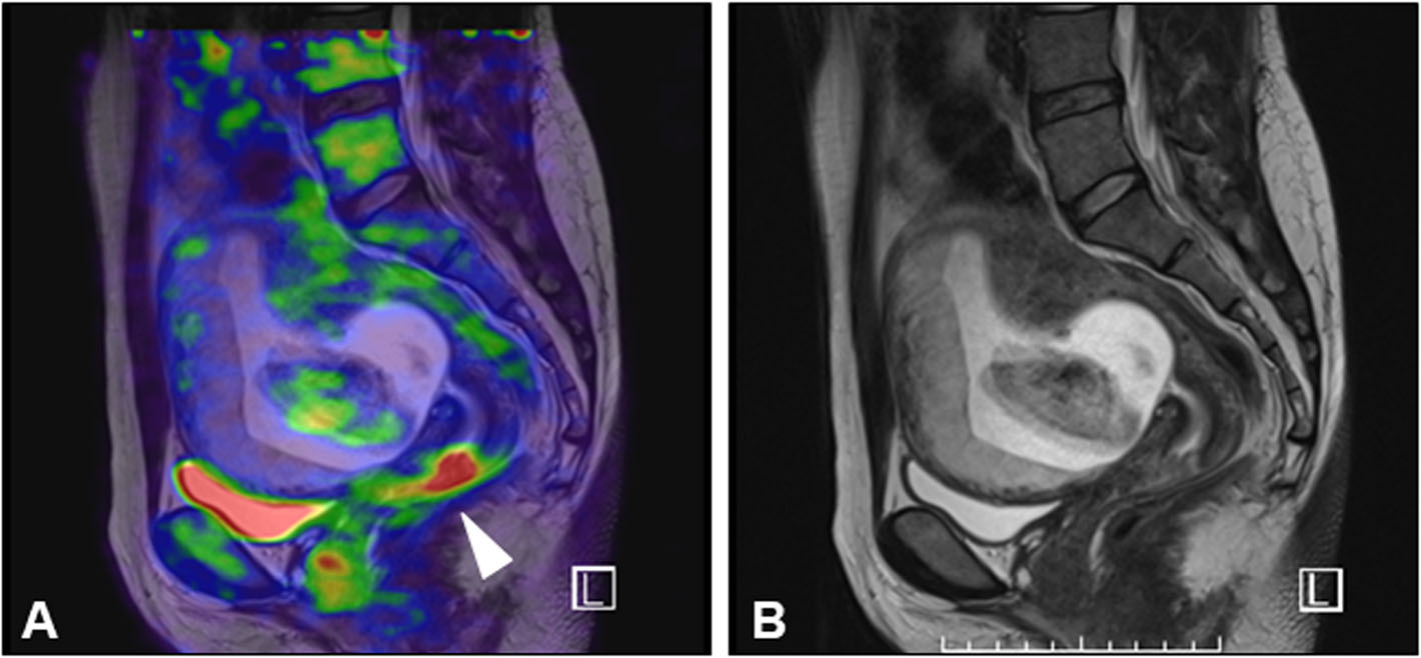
PET/MR images of case #3. a Sagittal view of PET/MR fusion image shows increased FDG uptake of cervical tumor (white arrowhead). b T2-weighted magnetic resonance image corresponding to PET/MR image (a)
